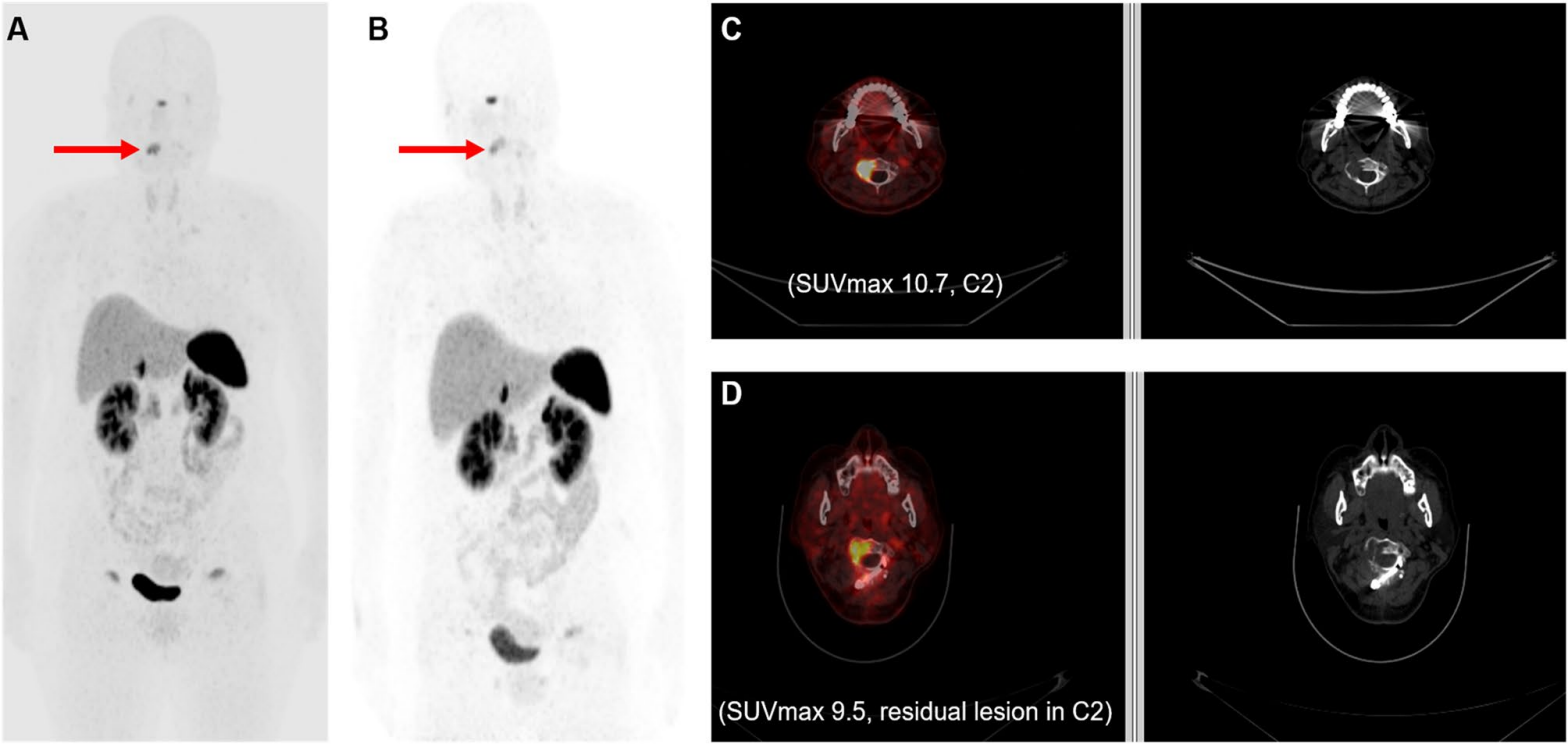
A case of unresolved hypophosphatemia after surgical excision was demonstrated by the follow-up with ^68^Ga-DOTATOC PET/CT. (A) MIP image of initial ^68^Ga-DOTATOC PET/CT with the red arrow indicating the culprit lesion; (B) MIP image of follow-up with ^68^Ga-DOTATOC PET/CT 6 months after surgery with the red arrow indicating the residual culprit lesion; (C) axial PET/CT and non-enhanced CT images of culprit lesion in initial ^68^Ga-DOTATOC PET/CT; (D) axial PET/CT image and non-enhanced CT images of residual culprit lesion in follow-up ^68^Ga-DOTATOC PET/CT.
